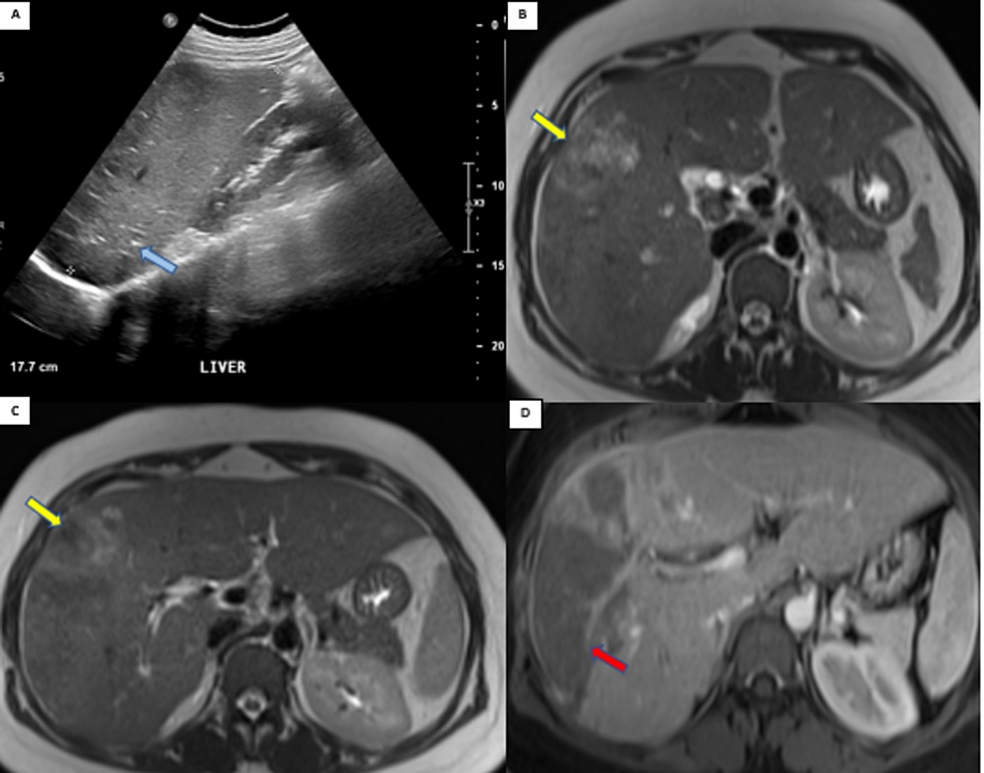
(A) Ultrasound abdomen showing enlarged liver with multiple calcifications (blue arrow). (B and C) MRI T2 haste showing large space-occupying lesion with heterogenous intermediate signal in segment 5 (yellow arrows) and (D) MRI T1 postcontrast showing enlarged liver with heterogeneous parenchymal enhancement and large non-enhancing lesion with septal enhancement (red arrow) in right lobe with multiple surrounding smaller ill-defined lesions.
